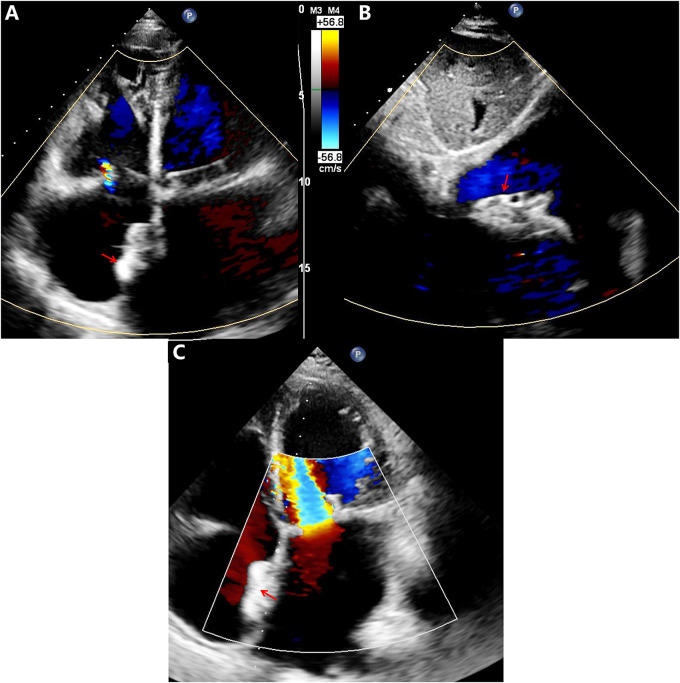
Postoperative and follow-up transthoracic echocardiography findings. (A,B) Color Doppler imaging in apical four-chamber and subcostal views demonstrates no residual interatrial shunt post-procedure; (C) Six-month follow-up confirms stable device position with complete elimination of interatrial flow.
